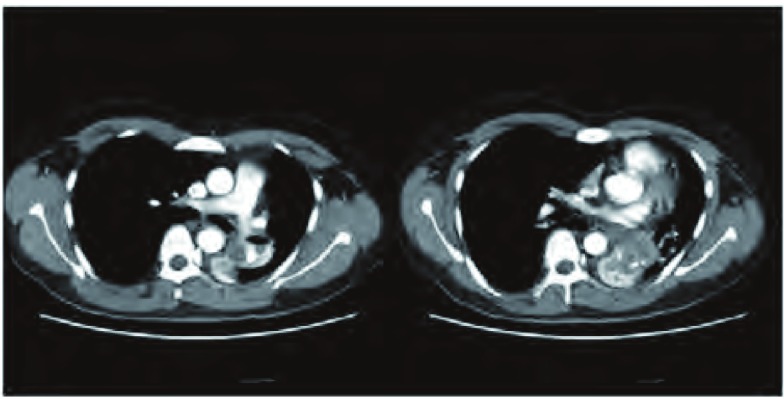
肺部CT：左肺下叶支气管开口处管腔内肿物及周围见团片状高密度影，伴左肺下叶肺不张。 Chest CT scan: A tumor is located in the left lower lobe bronchus openings and can see lamellar high-density shadow around it and atelectasis in the left lower lobe.
